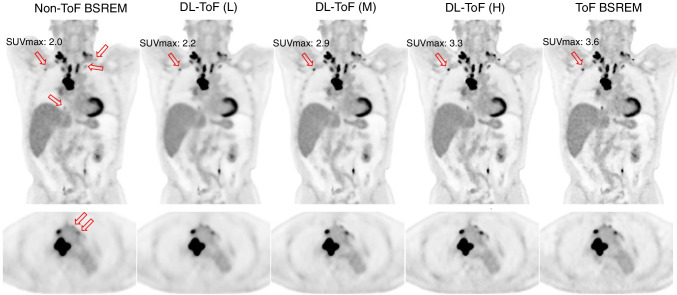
DL-ToF enhancement of a representative test subject with a BMI of 26.4 kg/m^2^ with an injected activity of 515 MBq scanned on GE Discovery MI (5-ring) PET/CT scanner (slice thickness 2.8 mm). Arrows point to lesions with lower detectability in non-ToF BSREM as well as the SUV_max_ of an example lesion (SUV_max_ values of all investigated lesions are summarised in Table 1 and shown in Fig. 3). Display window: 0–5 SUV
